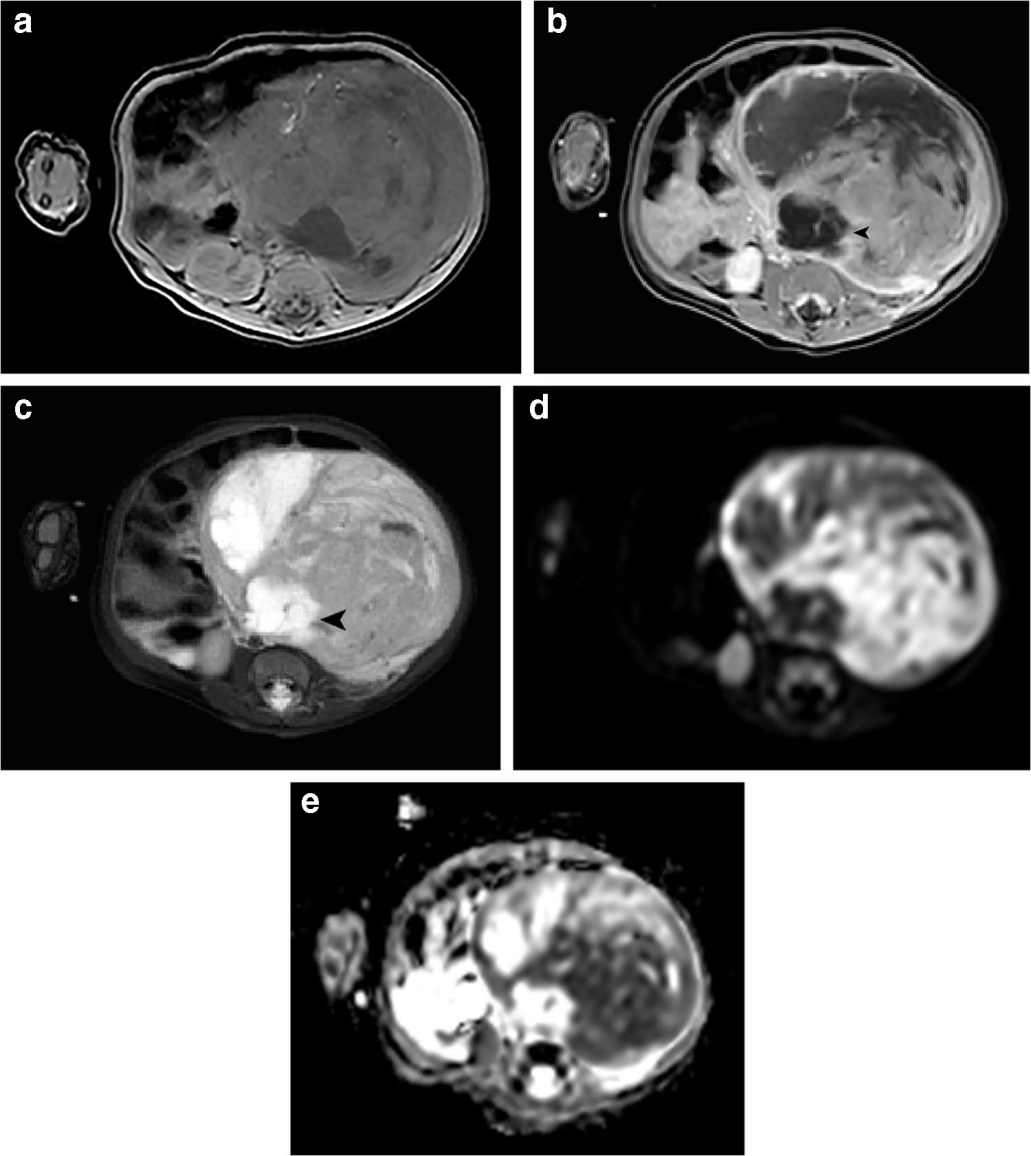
Magnetic resonance imaging of a 2-month-old girl with a left-sided cellular type congenital mesoblastic nephroma with a volume of 312 cm^3^. a Axial T1-weighted image shows a heterogeneous and isointense tumor. b Contrast-enhanced axial T1-weighted image shows heterogeneous enhancement, with some large cysts (*arrowhead*). c Axial T2-weighted image shows evident hemorrhage/necrosis and again isointensity of the solid components of the tumor, with hyperintense cysts (*arrowhead*). d On the axial diffusion weighted imaging b1000 sequence the tumor appears heterogeneous with varying diffusion restriction. e On the axial calculated apparent diffusion coefficient map, the solid parts of the lesion show considerable restricted diffusion compared to the (healthy) renal tissue, with a median apparent diffusion coefficient value of 1.000×10^−3^ mm^2^/s
